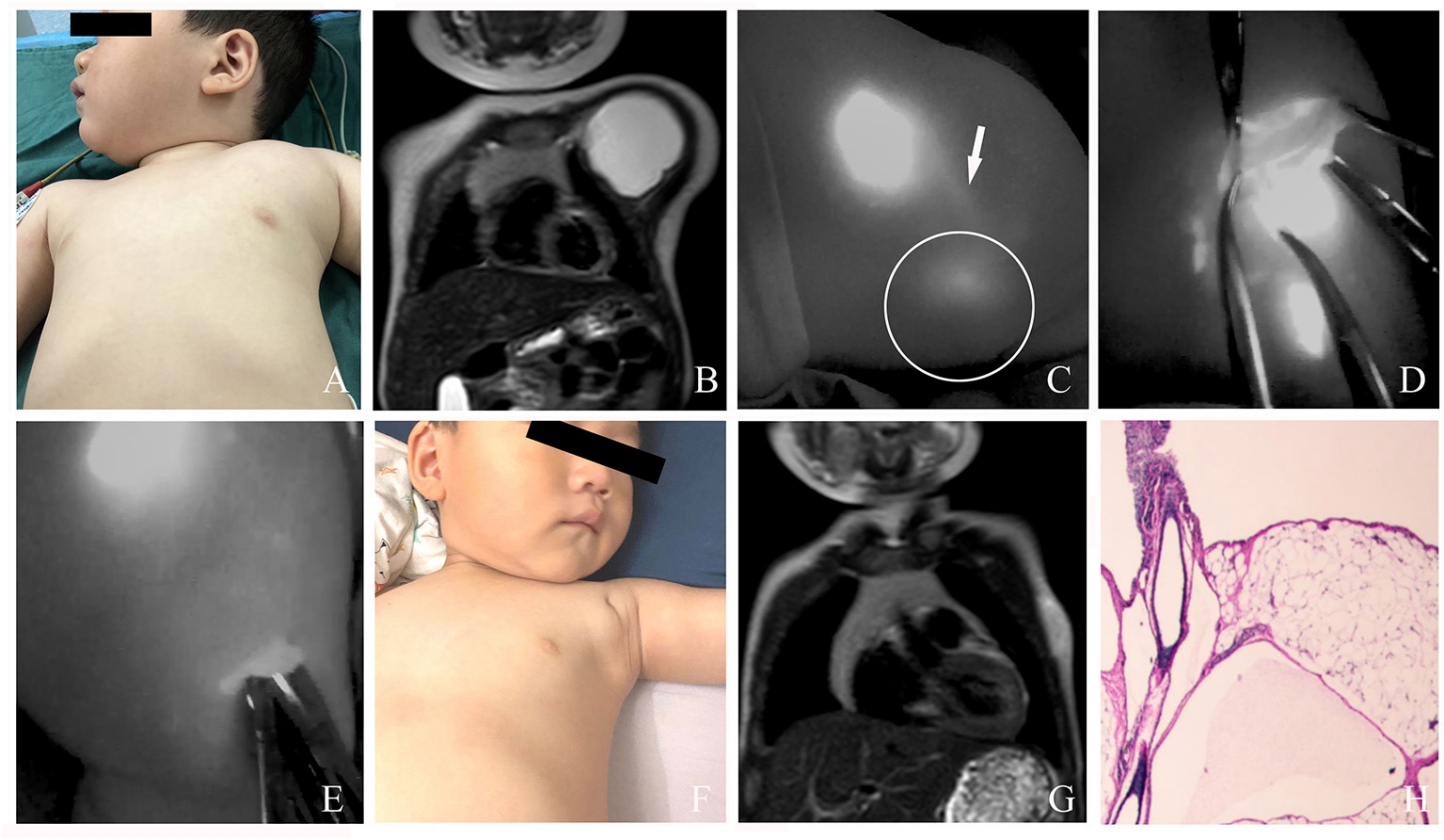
(A) A 2-year-old male patient with macro cLM of the left chest preoperatively. (B) MRI preoperatively. (C) ICG finding with one afferent lymph vessel draining into a lesion. (D) The cLM lesion confirmed by ICG lymphography during surgery. (E) No inflow visualization or lymphatic leak on the previous detected site after inflow occlusion. (F) Ten months postoperatively, complete regression of cLM met the criterion of excellent curative effect. (G) MRI postoperatively. (H) Pathology postoperatively. *Arrow head*, lymph vessel; *circle*, region of cLM.
